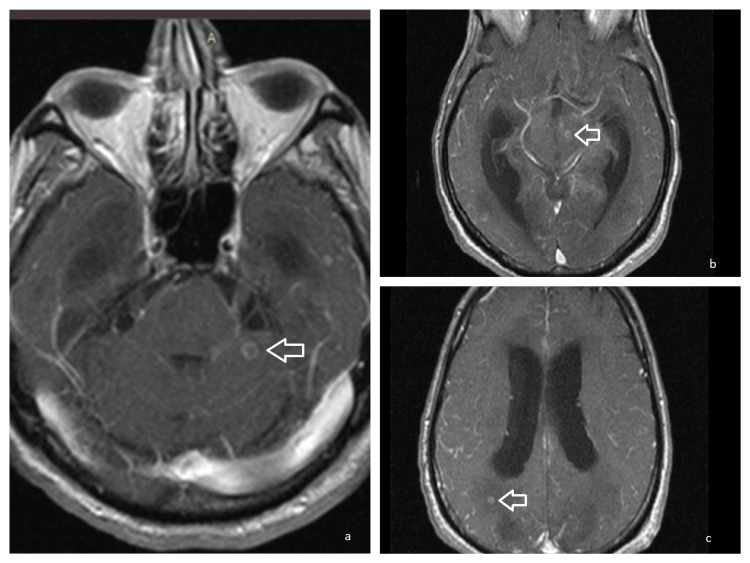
MRI of the brain T1WI Ring enhancing lesions (arrows) of the left anterior lobe of the cerebellum (a), the midbrain (b), and the right parietal lobe (c), typical for tuberculomas. T1WI: T1-weighted imaging
